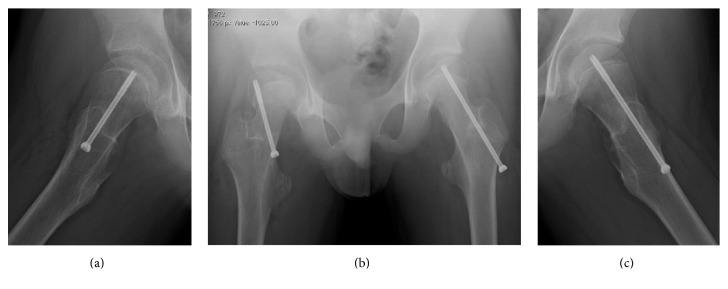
Postoperative (a) frog-lateral radiograph of the right hip joint. (b) Anteroposterior radiograph showing the screw for the valgus SCFE was placed more medially than for a typical varus SCFE. (c) Frog-lateral radiograph of the left hip joint.
